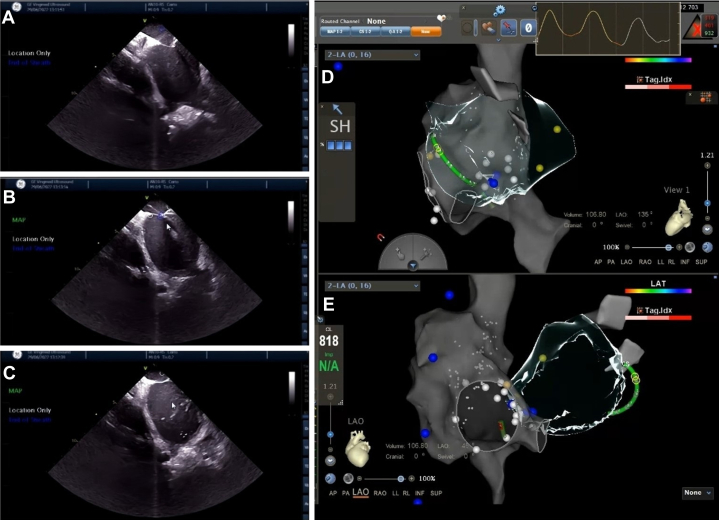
Zero-Fluoroscopy Pericardiocentesis Guided by ICE and EAM via CARTO3 System (A) ICE reveals the “tenting-like sign”; (B) transseptal puncture is executed under real-time ICE guidance; (C) the success of the puncture needle entering the left atrium is confirmed by injecting saline through the needle lumen; (D and E) EAM was used to create a detailed reconstruction of the right atrium, both venae cavae, and the interatrial septum. EAM = electroanatomic mapping; ICE = intracardiac echocardiography.2.Pre-preparation of pericardial puncture ICE was used to trace the epicardial boundaries of the left and right ventricles, including the lateral walls, apex, and ventricular septum. These structures were mapped to their corresponding epicardial surfaces, and an epicardial anatomical model was created using CARTOSOUND (Figure 6).
